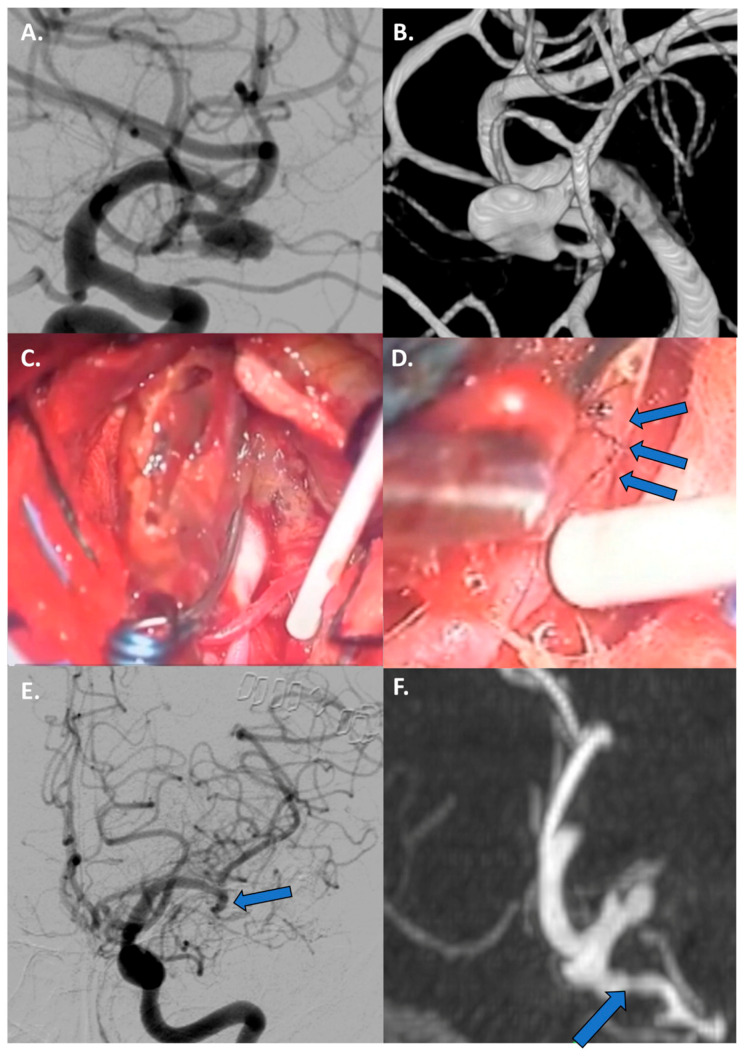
Case 3: Thrombectomy with bypass in a giant, fusiform, and partially thrombosed 21 mm M2 aneurysm carried by a 68-year-old woman with a history of seizures. (A,B) Preoperative DSA with 3D reconstruction reporting the giant, fusiform, and partially thrombosed aneurysm at left M2. (C) Proximal control of M2 performed with a temporary clipping and progressive debulking of intrasaccular thrombus using cavitronic ultrasonic surgical aspirator until visualization of the ostia (blue arrows). (D) An intracranial end-to-side bypass between left temporal M3 branch and distal M2 branch. (E) DSA confirming bypass flow and flow replacement (blue arrows). (F) A 6-month follow-up CTA documenting the complete exclusion of the aneurysm (blue arrows).
